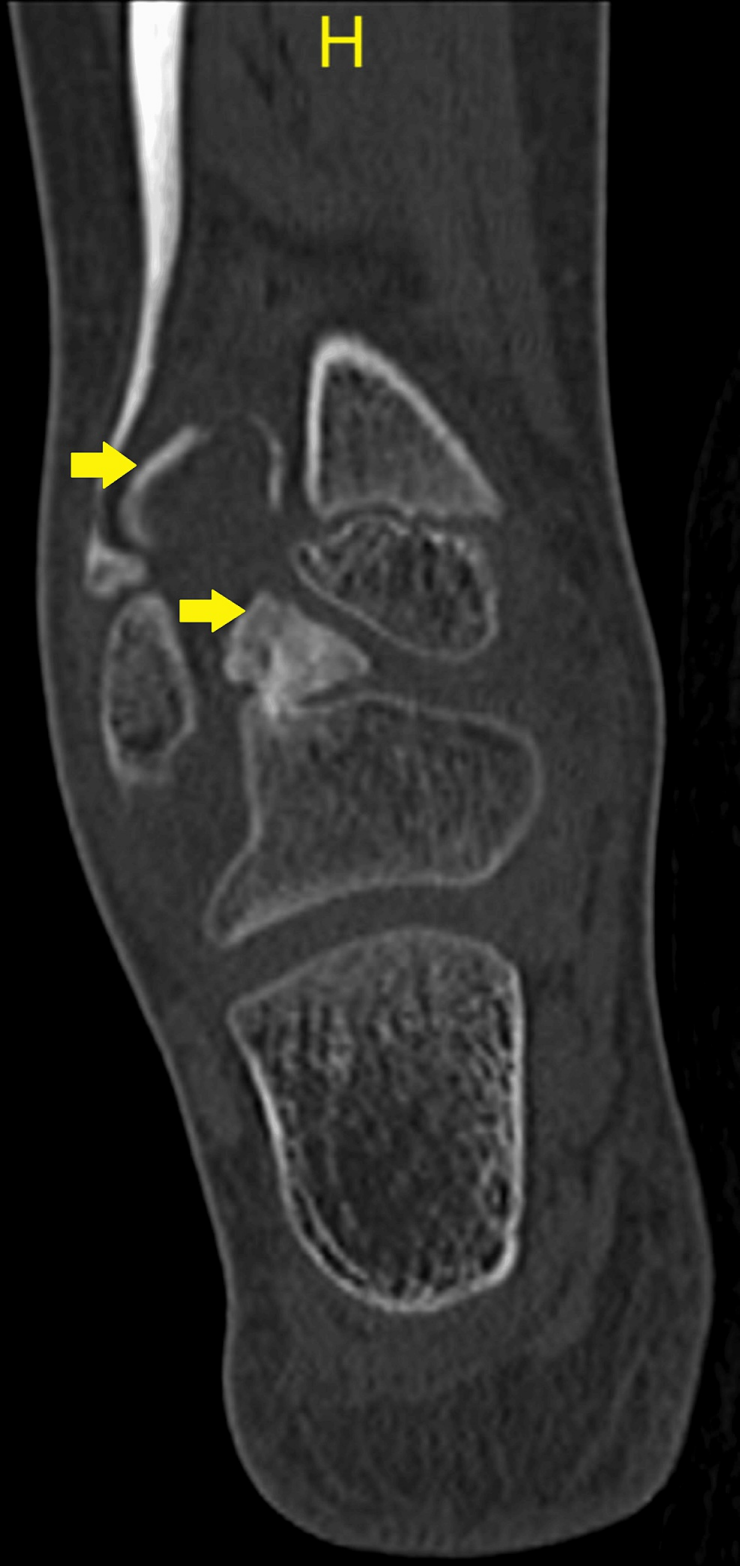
CT coronal image: arrows demonstrate the osteochondromas from the posterior aspect of the talus and in the interosseous region
